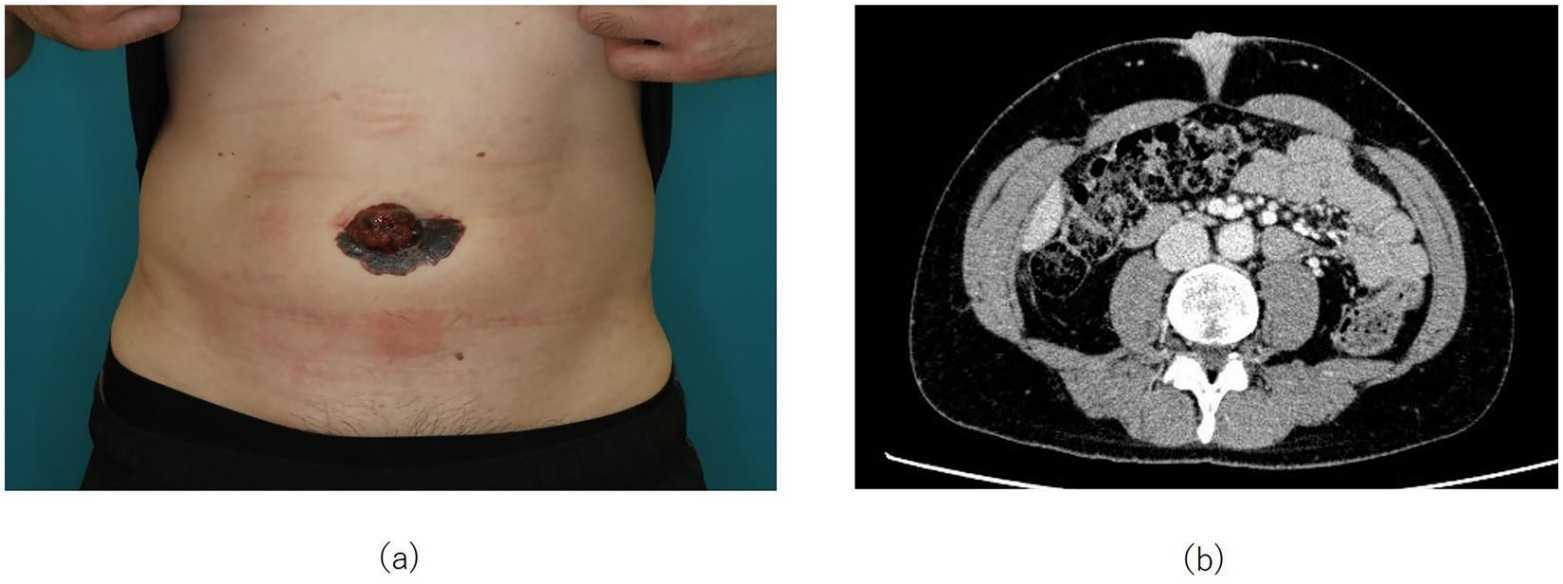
Initial presentation at our department. (a) Clinical photograph. A 67 × 32 mm black macule is observed at the umbilicus, with a 38 × 22 mm reddish nodule centrally located within the lesion. (b) Contrast-enhanced axial CT image. The tumor shows invasion extending to the fascia.
